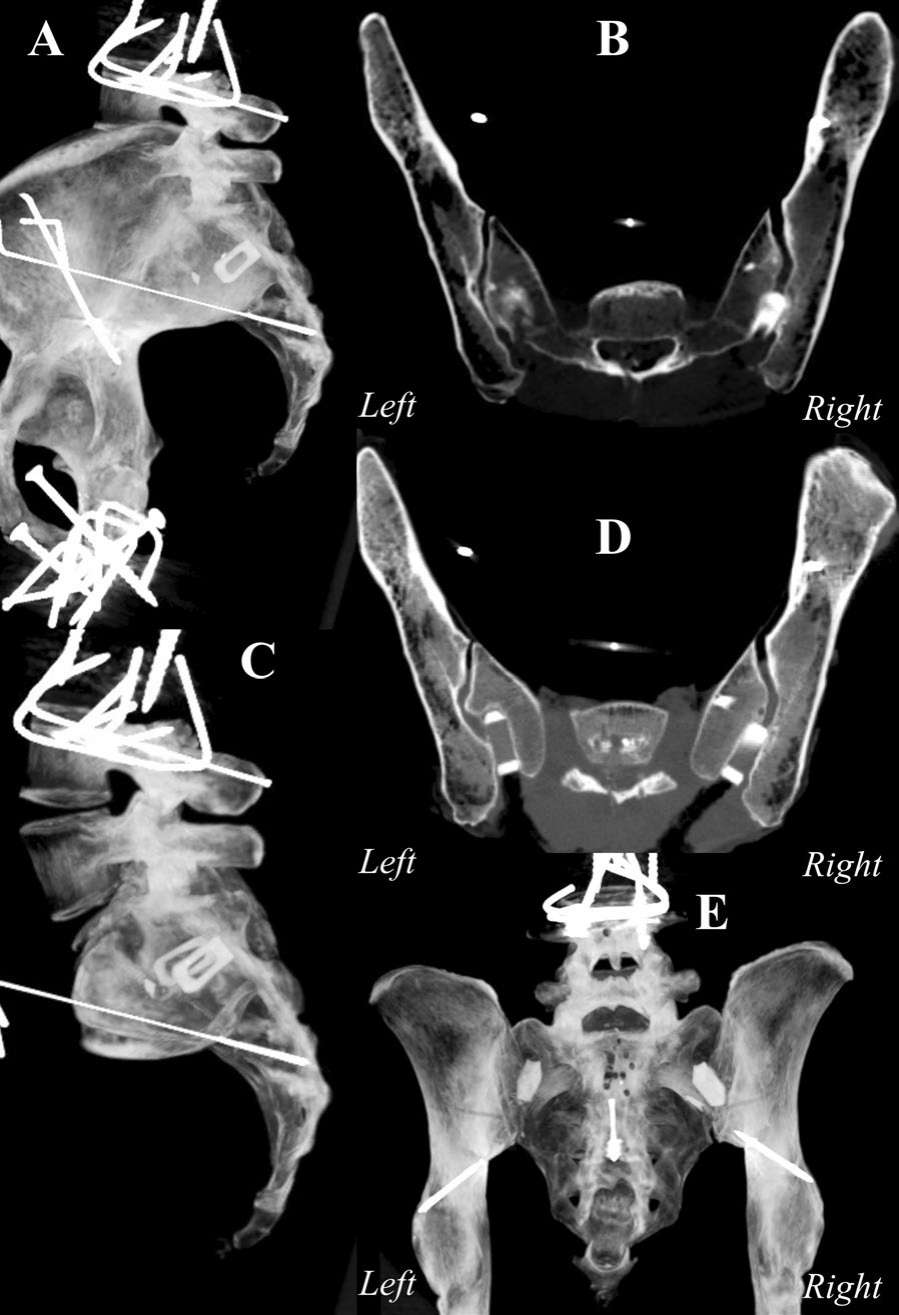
Maximum intensity projection (MIP) renderings, and images obtained from postoperative computed tomography (CT) scans upon fixation with bone allograft implant. Images are displayed are A sagittal pelvis [unilateral fixation], B axial pelvis [unilateral fixation], C sagittal sacrum only [bilateral fixation], D axial pelvis [bilateral fixation], E coronal pelvis [bilateral fixation]
